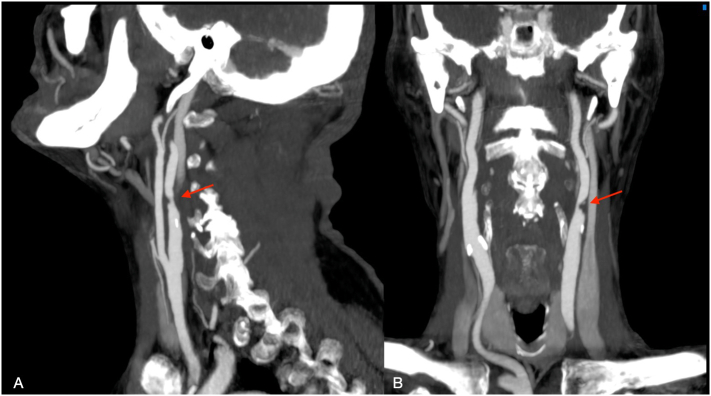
Preoperative CT Angiography showing stenosis of left internal carotid artery in sagittal plane (A) and coronal plane (B).
